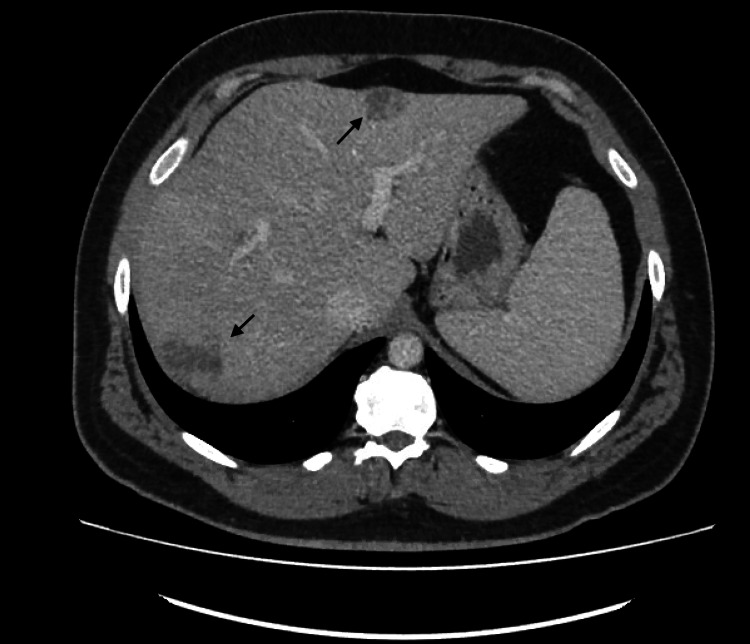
CT abdomen CT abdomen showing lesions on the anterior (arrow above) and inferior (arrow below) aspects of the right lobe of the liver
